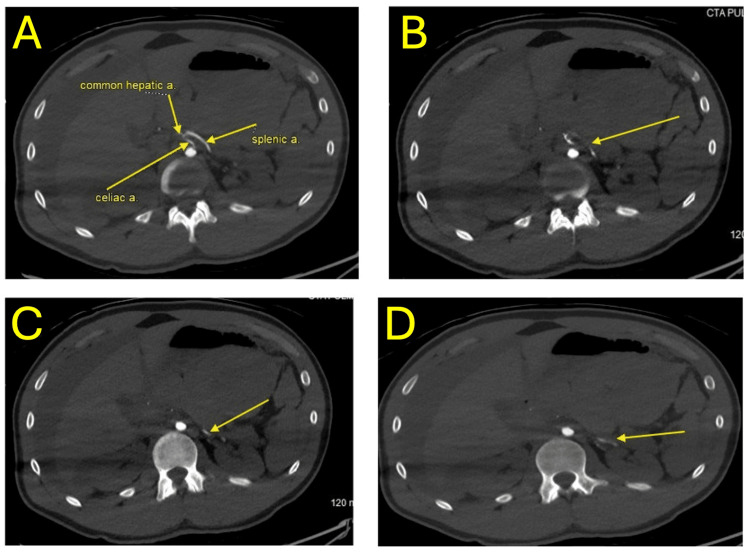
Axial CTA Demonstrating Hemorrhagic Ascites Without an Identifiable Vascular Source Axial computed tomography angiography (CTA) images demonstrating intraperitoneal fluid concerning for hemorrhagic ascites without definitive evidence of active contrast extravasation or visceral artery aneurysm. (A) Celiac axis with labeled common hepatic artery and splenic artery. (B–D) Sequential axial images demonstrating the course of the splenic artery (arrows) without evidence of aneurysmal dilation or active contrast extravasation.
